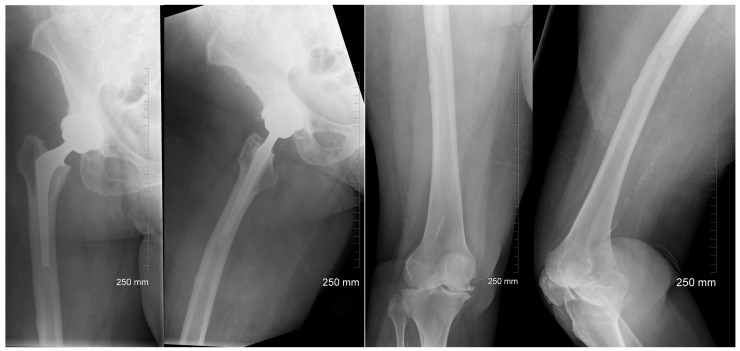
Radiographs of contralateral right femur demonstrating lateral cortical thickening distal to stem of femoral implant in 78-year-old female patient.
